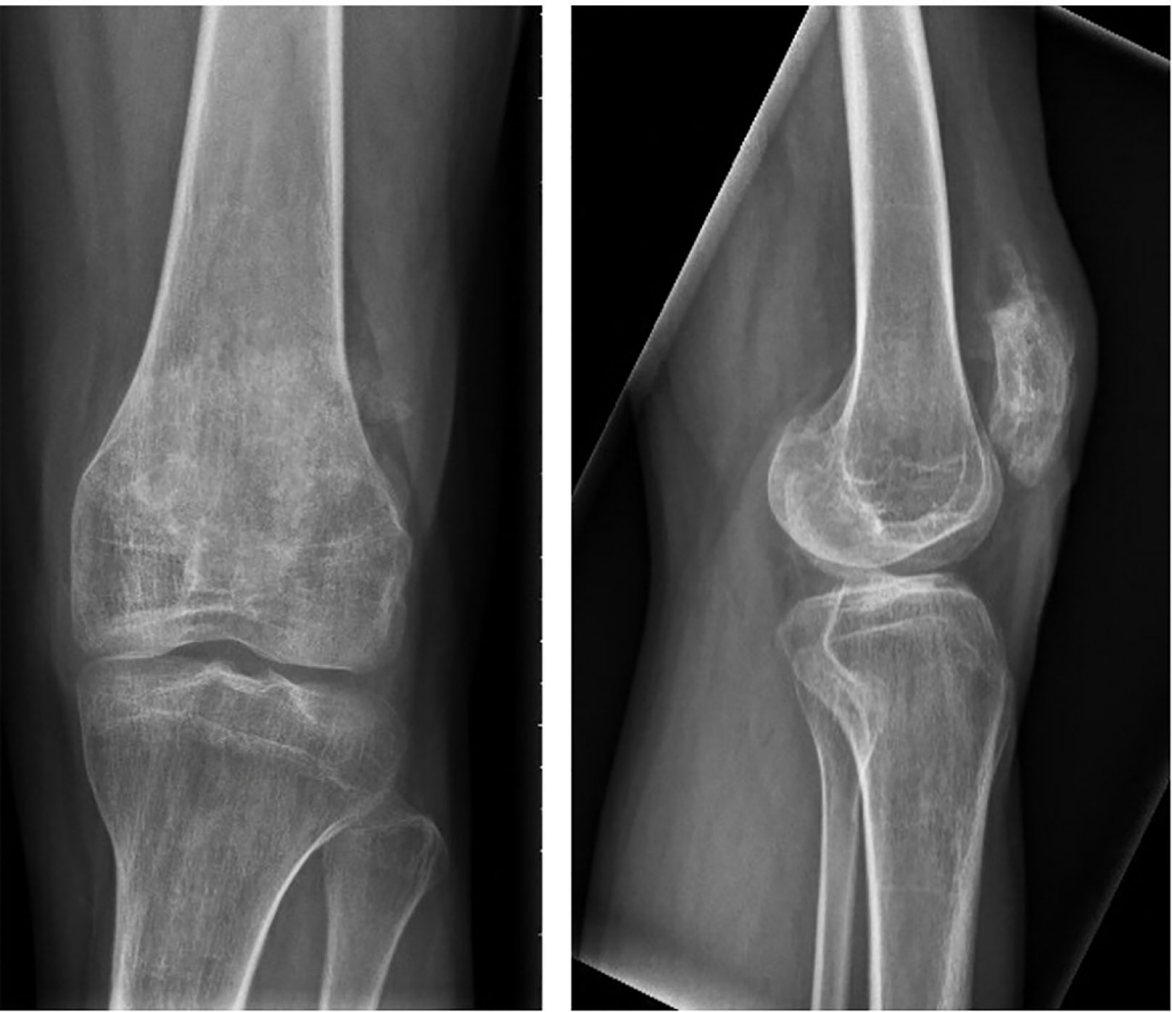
AP and lateral radiographs at 3 months, showing healing of the transverse left patella fracture.
